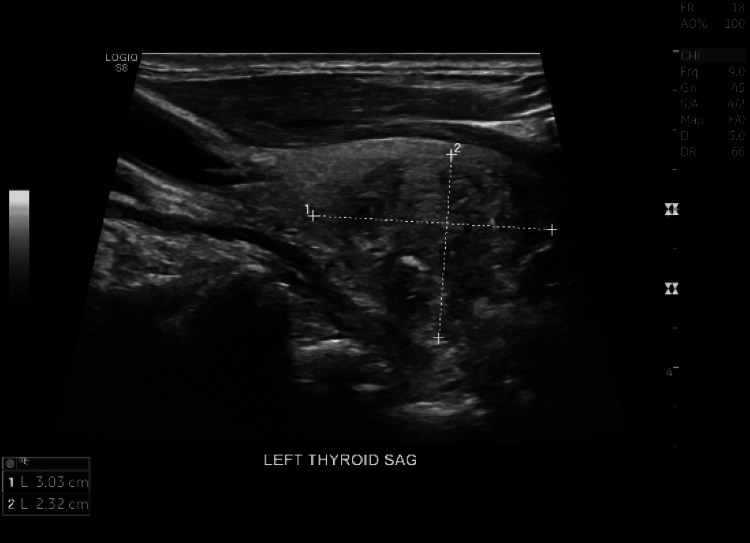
Ultrasound thyroid showing the mass occupying the left mid to lower pole, measuring 3.0 x 1.9 x 2.3 cm
